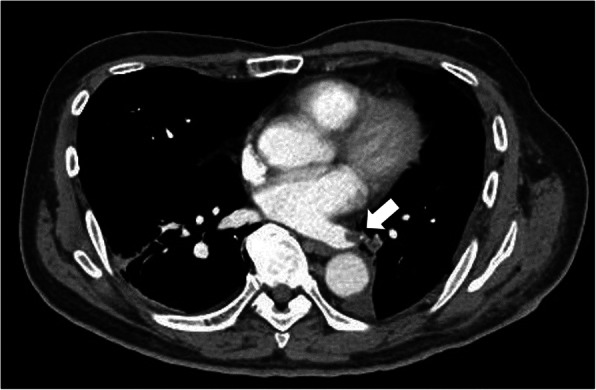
Contrast-enhanced computed tomography image of the chest taken at the onset of acute limb ischemia. The arrow indicates the left inferior pulmonary vein stump thrombus
